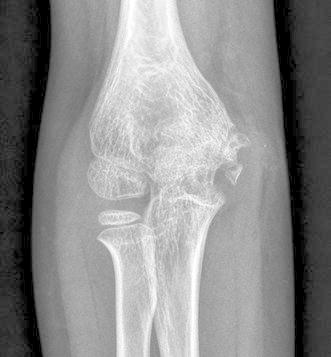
Follow-up at 3 years 2 months: anteroposterior radiograph shows an irregular, speculated bony trochlea and a normal Baumann angle (15°)
